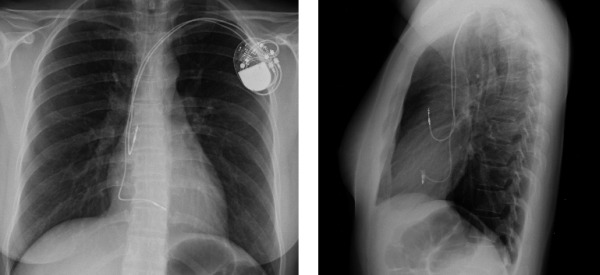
Chest x-ray demonstrates adequate position of the atrial and ventricular leads after delivery of pregnancy
